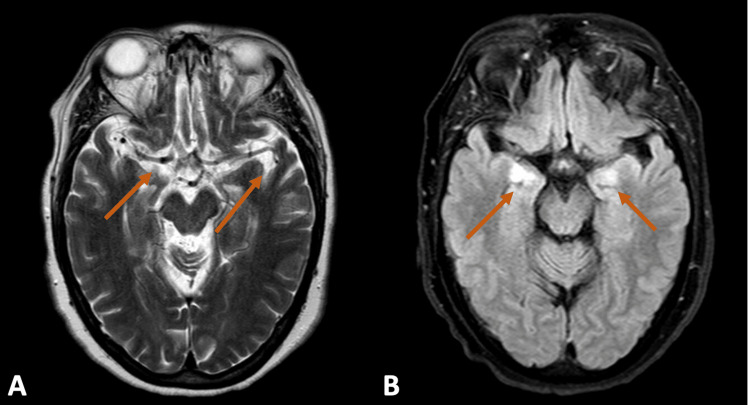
Patient's brain MRI A: Axial T2 shows a bilateral hyperintensity of the temporal lobes. B: Axial T2 FLAIR showing hyperintensity in the medial temporal lobe bilaterally.
